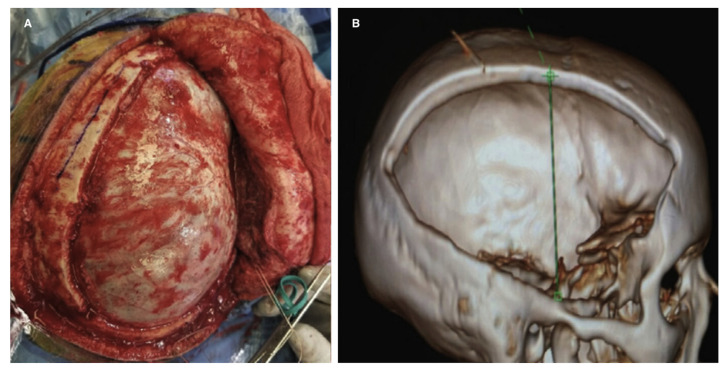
Panel (A) shows an intraoperative view of a wide frontal-temporal-parietal decompressive craniectomy before dural opening. Panel (B) displays a three-dimensional CT reconstruction demonstrating effective decompression of the anterior and middle cranial fossae, with adequate release of the temporal floor and frontal base to achieve meaningful intracranial volume expansion.
